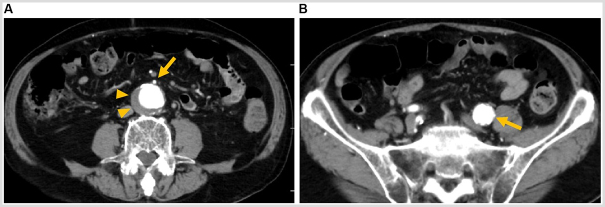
Preoperative abdominal contrast-enhanced computed tomography images. (A) A 39-mm abdominal aortic aneurysm (arrowheads) is observed caudally to the branching point of the inferior mesenteric artery (arrow). (B) A 25-mm left common iliac artery aneurysm is observed immediately cranial to the bifurcation of the internal and external iliac arteries (arrow).
